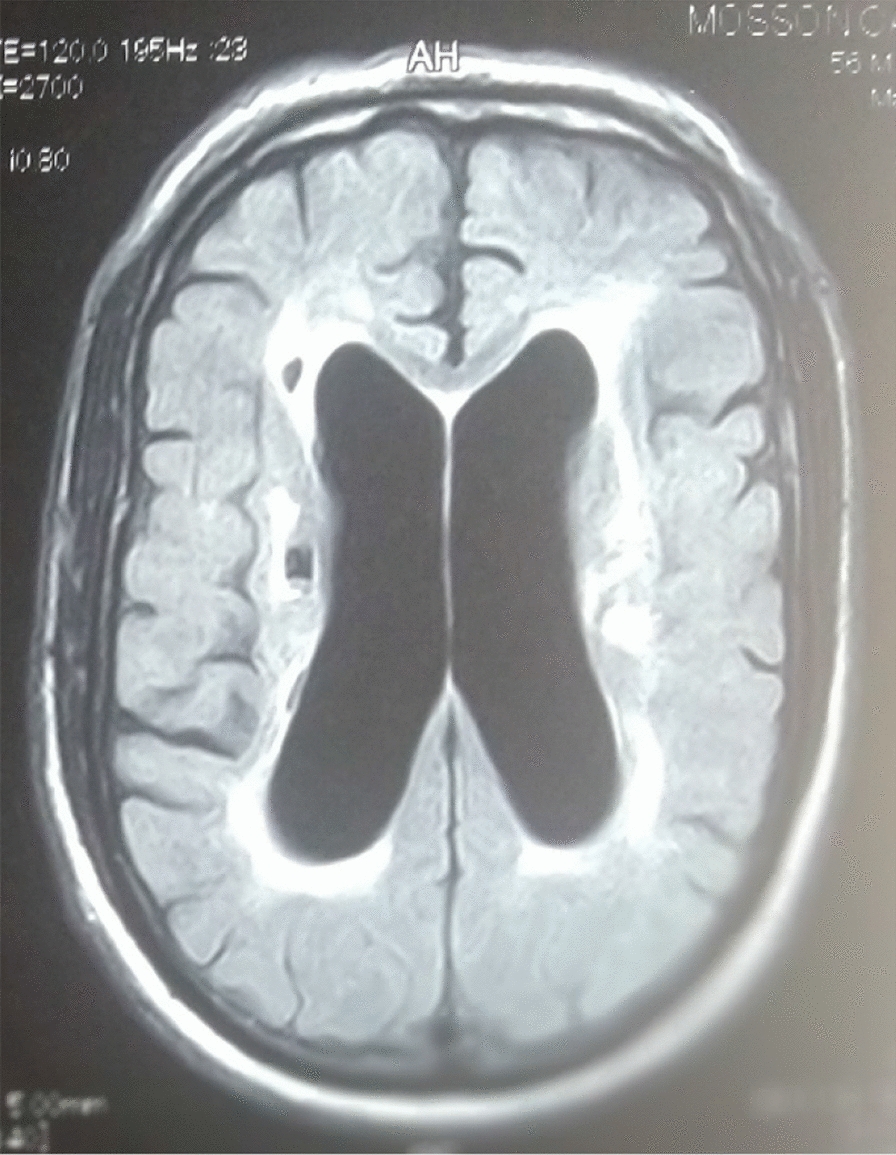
Cerebral MRI: focal abnormality in subcortical white matter, consisting of hyperintense signal on T1-weighted image
